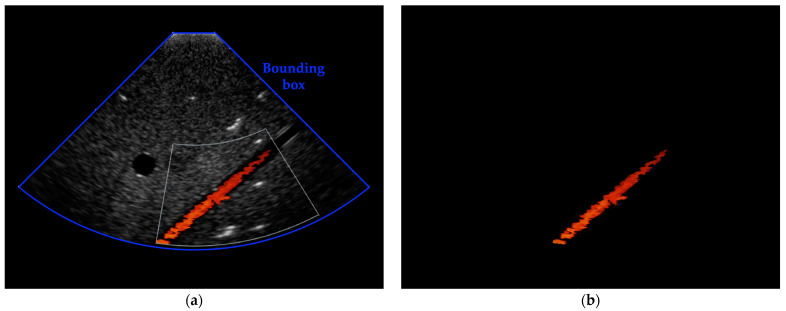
(a) Example of bounding box extraction in the first sampled frame before threshold-based filter application; (b) corresponding filtered frame containing the color Doppler information only.
